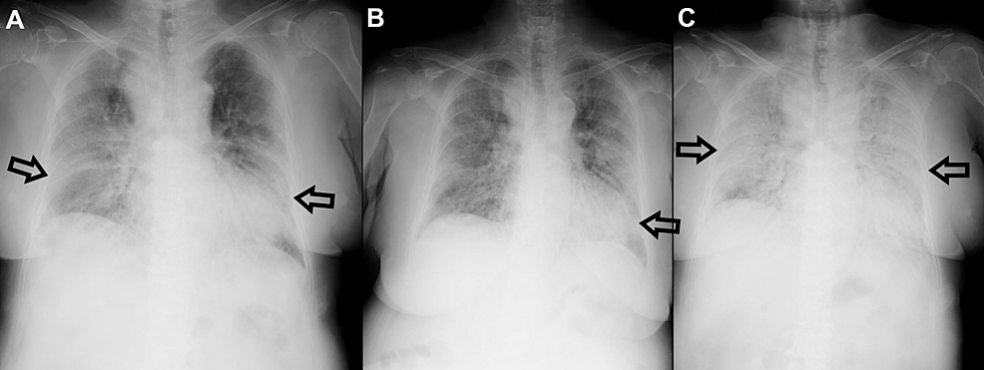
Chest radiographs (A) Day 2, (B) day 5, and (C) day 7.
